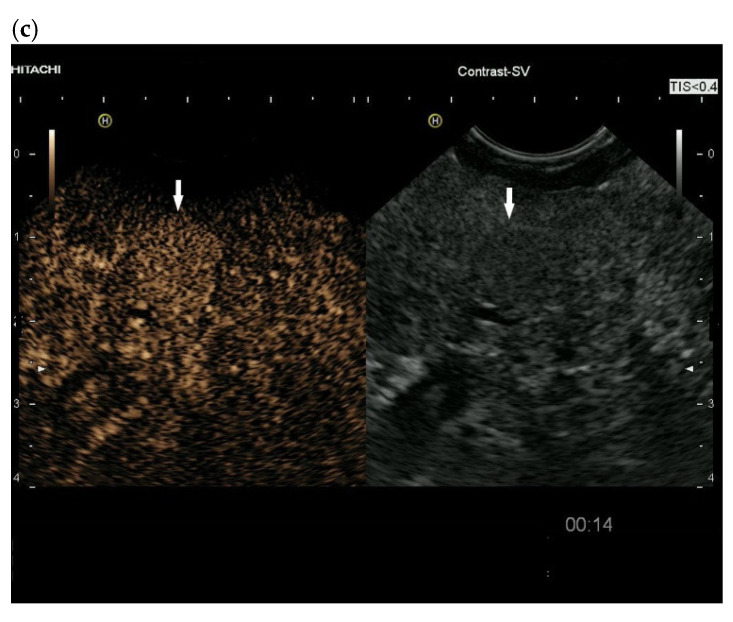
Intrapancreatic metastasis of an RCC. Male, 69 years old, with a small lesion in the body of the pancreas on surveillance MRI. Tumor nephrectomy 17 years ago, pancreatic tail resection with splenectomy for intrapancreatic metastasis 7 years ago. Endosonographically 10 mm, slightly hypoechoic lesion in the body of the pancreas. No evidence of vessels in the lesion in the native duplex EUS (a). In the EUS strain elastography, the lesion is not stiffer, rather soft (b). In the CH-EUS with 4.8 mL SonoVue, the lesion in the arterial phase shows homogeneous hyperenhancement. The arrow marks the hyperenhanced lesion in the left image, and an arrow marks the lesion in B-mode US on the right. (c). The RCC metastasis was diagnosed using EUS-FNP. The patient underwent pancreatic resection for second time.
